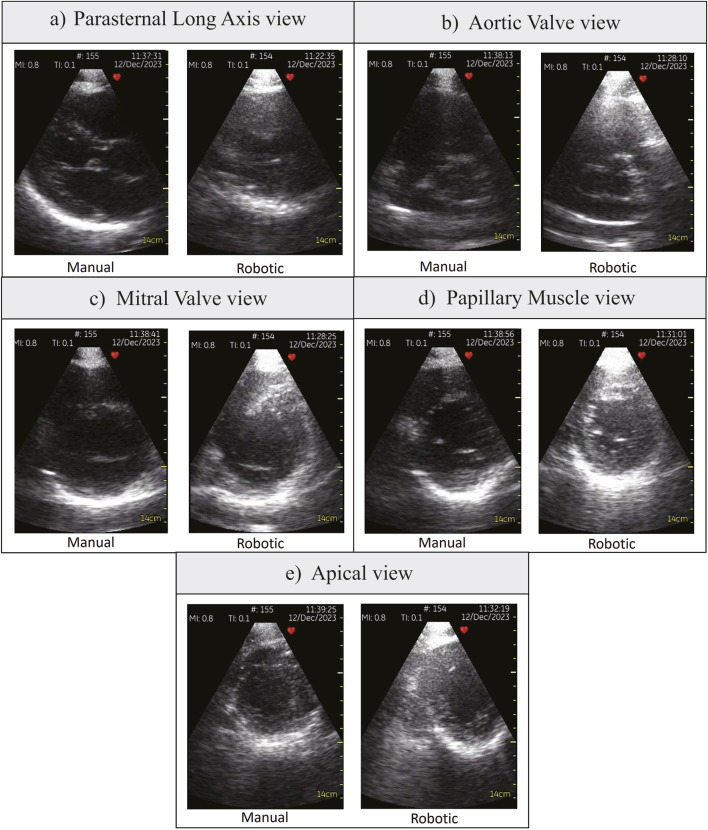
Various parasternal views obtained by participant P4, a doctor. (A) Parasternal Long Axis view, (B) Aortic Valve view, (C) Mitral Valve view, (D) Papillary Muscle view, (E) Apical view.
